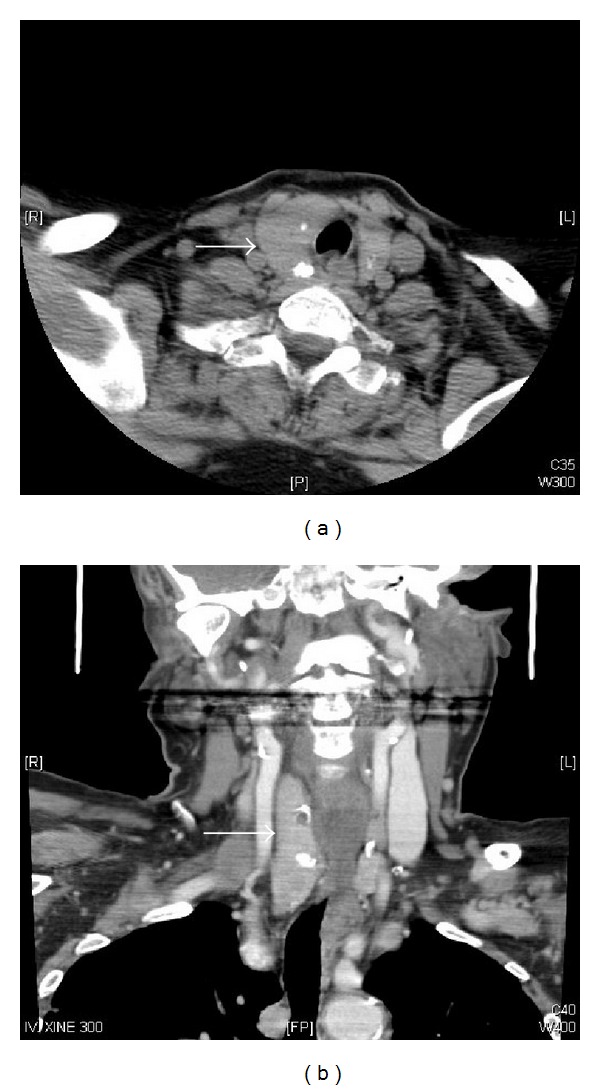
Computed tomography of neck showing diffuse enlargement of right lobe of thyroid, came out as papillary follicular cell variant thyroid carcinoma pT2N1.
